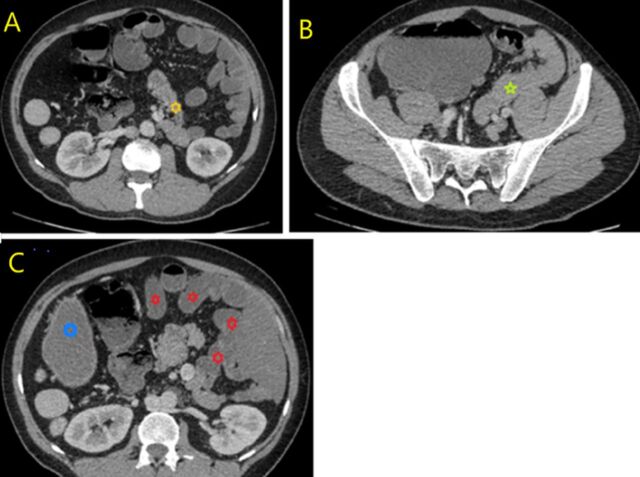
Intestinal malrotation without volvulus. Contrast-enhanced axial fat-saturated computed tomography image shows (A) abnormal position of the duodenojejunal junction (yellow asterisk), (B) jejunal loops located predominantly in the left lower quadrant (green star), and (C) ileal loops located predominantly in the left upper quadrant (red asterisks) with the stomach in the right quadrant (blue circle).
